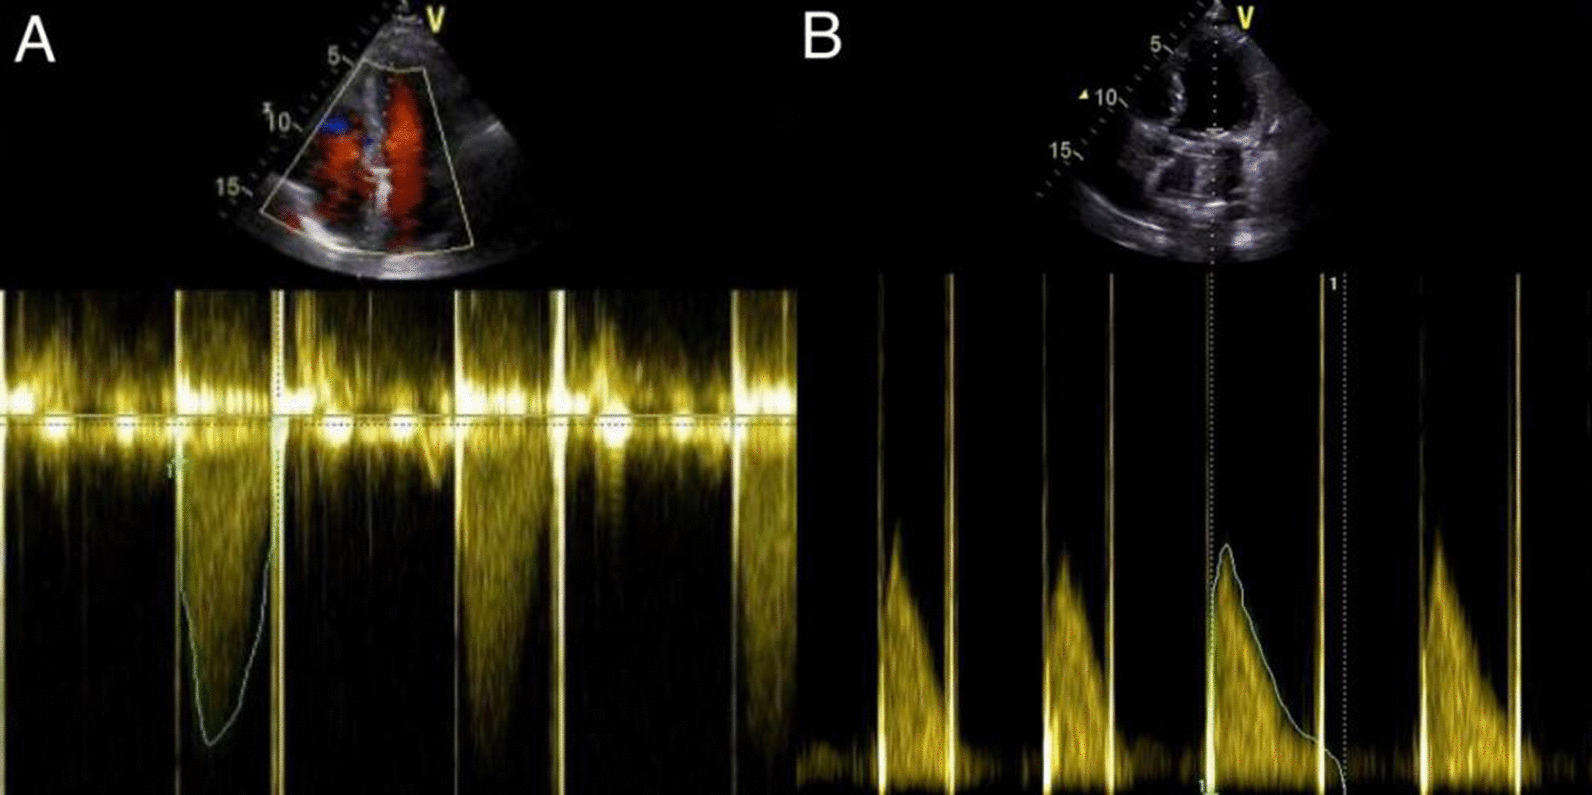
Post-operative echocardiographies of the patients with aortic valve and mitral valve replacements. A The echocardiography after aortic valve replacement shows that the forward blood flow is normal, with no obvious regurgitation or perivalvular leakage; B The echocardiography after mitral valve replacement shows that the forward blood flow is normal without obvious regurgitation or perivalvular leakage
